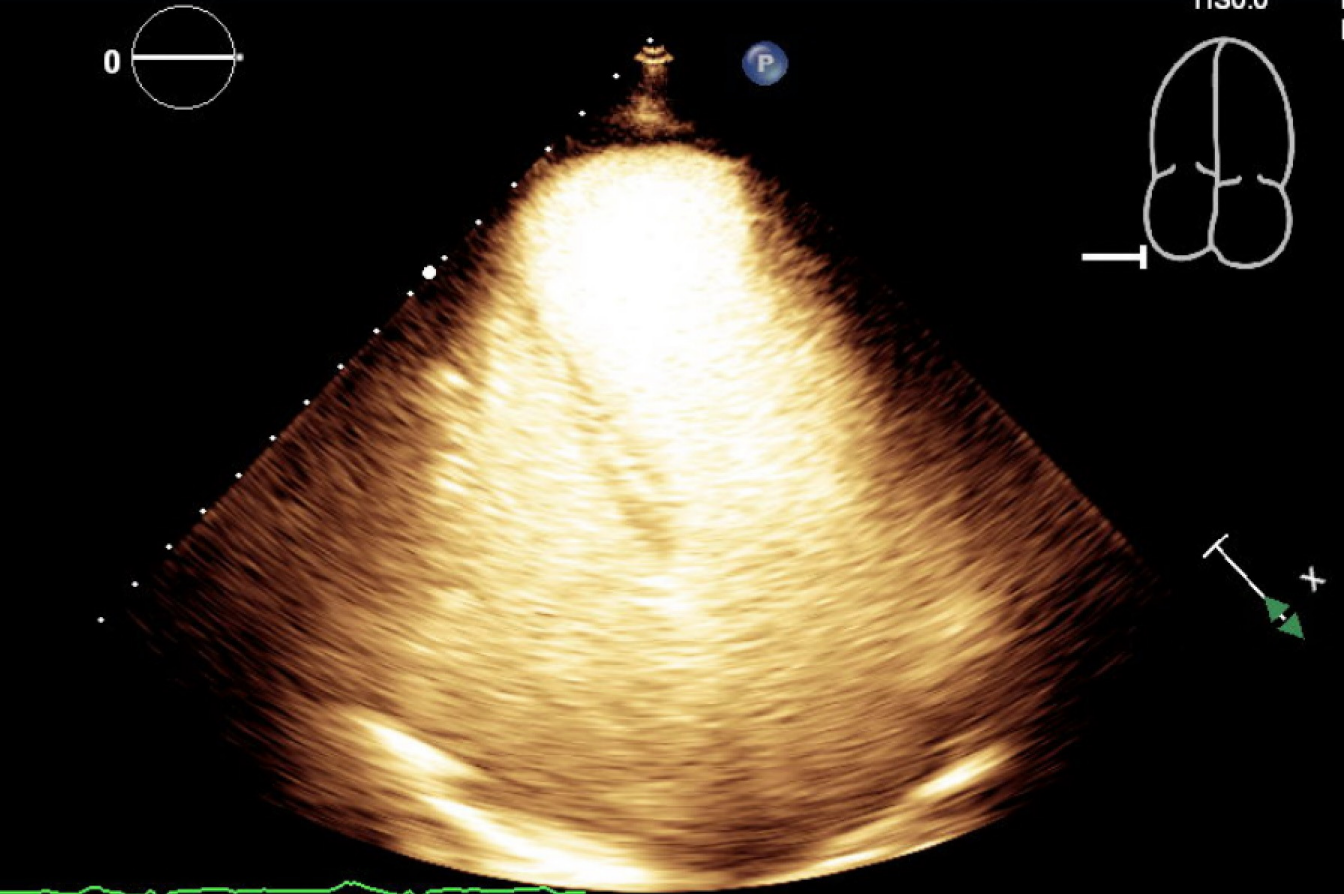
TTE with contrast at the apical 4 chamber view shows resolution of LV thrombi. TTE, Transthoracic echocardiogram; LV, left ventricular
